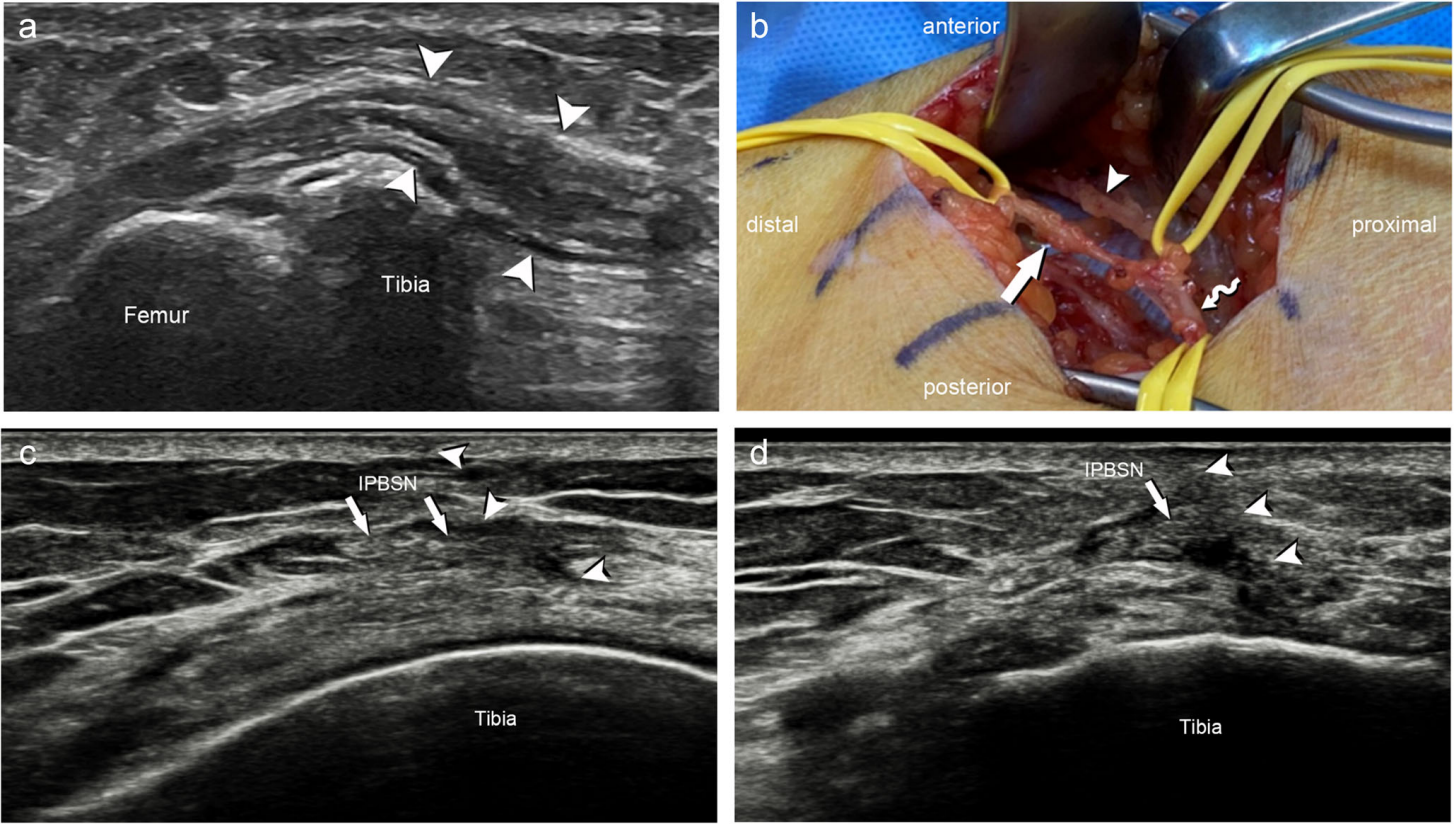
a Neuroma-in-continuity of the infrapatellar branch of the saphenous nerve (IPBSN). Longitudinal sonogram shows diffuse hypoechoic enlargement of the inferior branch of the IPBSN (arrowheads) with loss of fascicular architecture. b Intraoperative photography of the corresponding neuroma-in-continuity of the inferior branch of the IPBSN (arrow). The IPBSN (wavy arrow) and the superior branch of the IPBSN (arrowhead) are unremarkable. c, d Entrapment of the inferior branch of the IPBSN (arrows) due to scar tissue (arrowheads) at the level of the tibial tuberosity on transverse (c) and longitudinal (d) transducer positions
